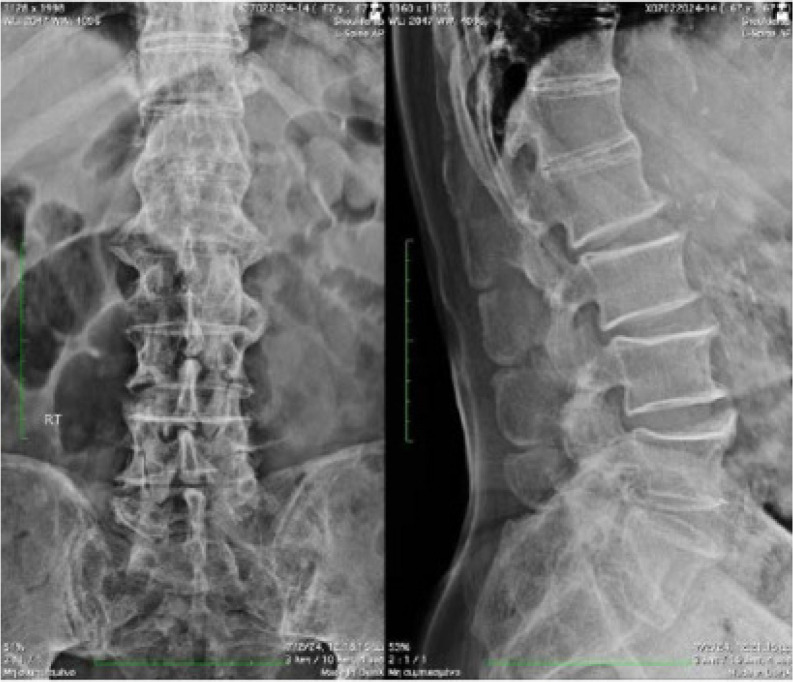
Lumbar spine x-rays show hypertrophic changes and excess bridging osteophytes between the vertebral bodies.
